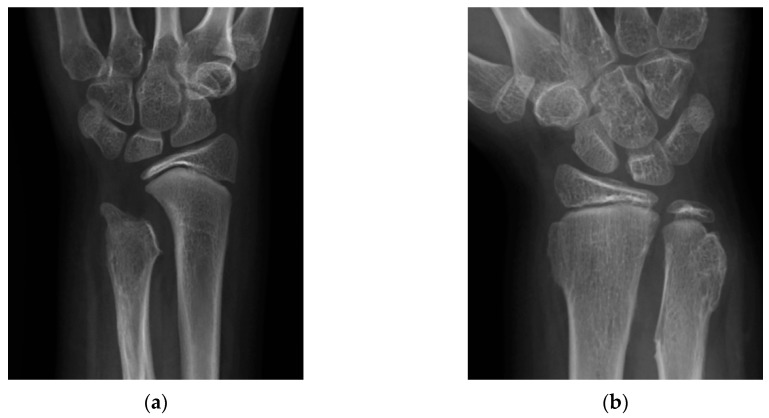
Radiographs of (a) a 10-year-old girl who underwent ulnar lengthening with recurrence two years later showed loss of the discoid nature of the distal ulnar physis; (b) a 9-year-old boy following ulnar lengthening at the age of three without recurrence showed smooth discoid nature of distal ulnar physis.
